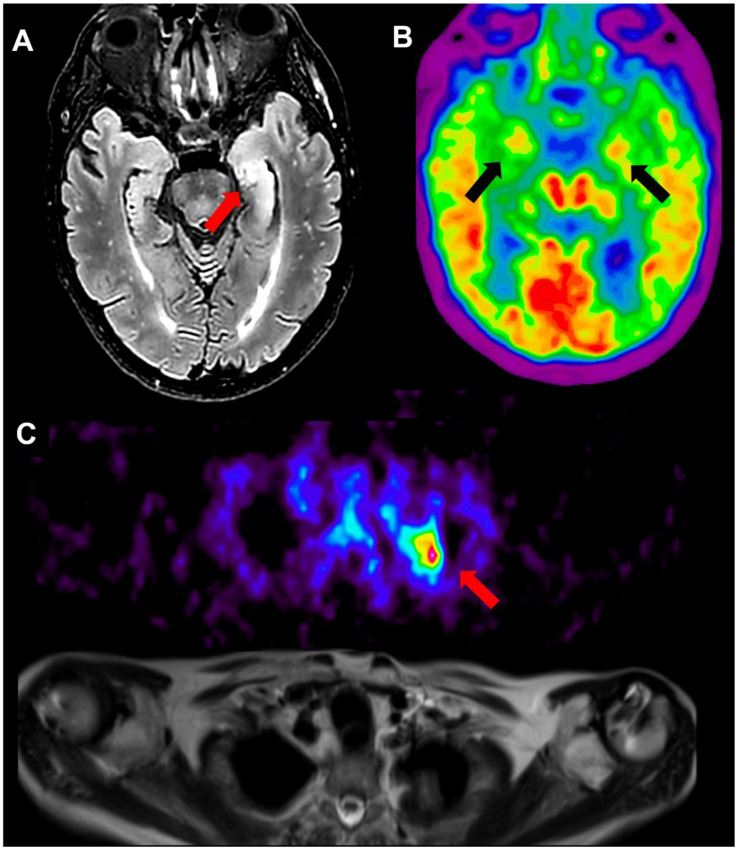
(A) T2 Flair MRI demonstrating increased T2 signal in left hippocampus; (B) [18F]FDG PET showing bilateral increased uptake in amygdala and portions of hippocampus; (C) [18F]FDG PET (upper) and T2 Haste MRI (lower) showing left apical lung neoplasm.
